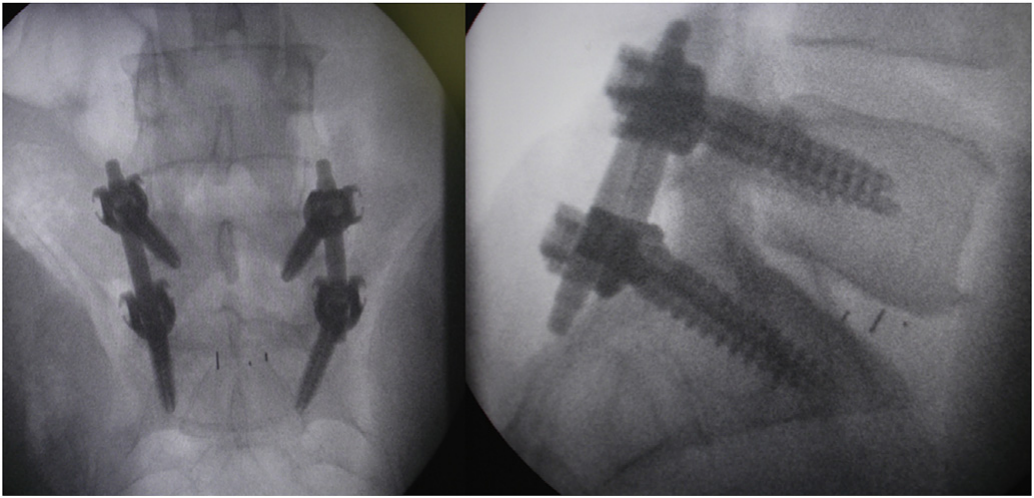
Intraoperative, x-ray confirming hardware placement.
